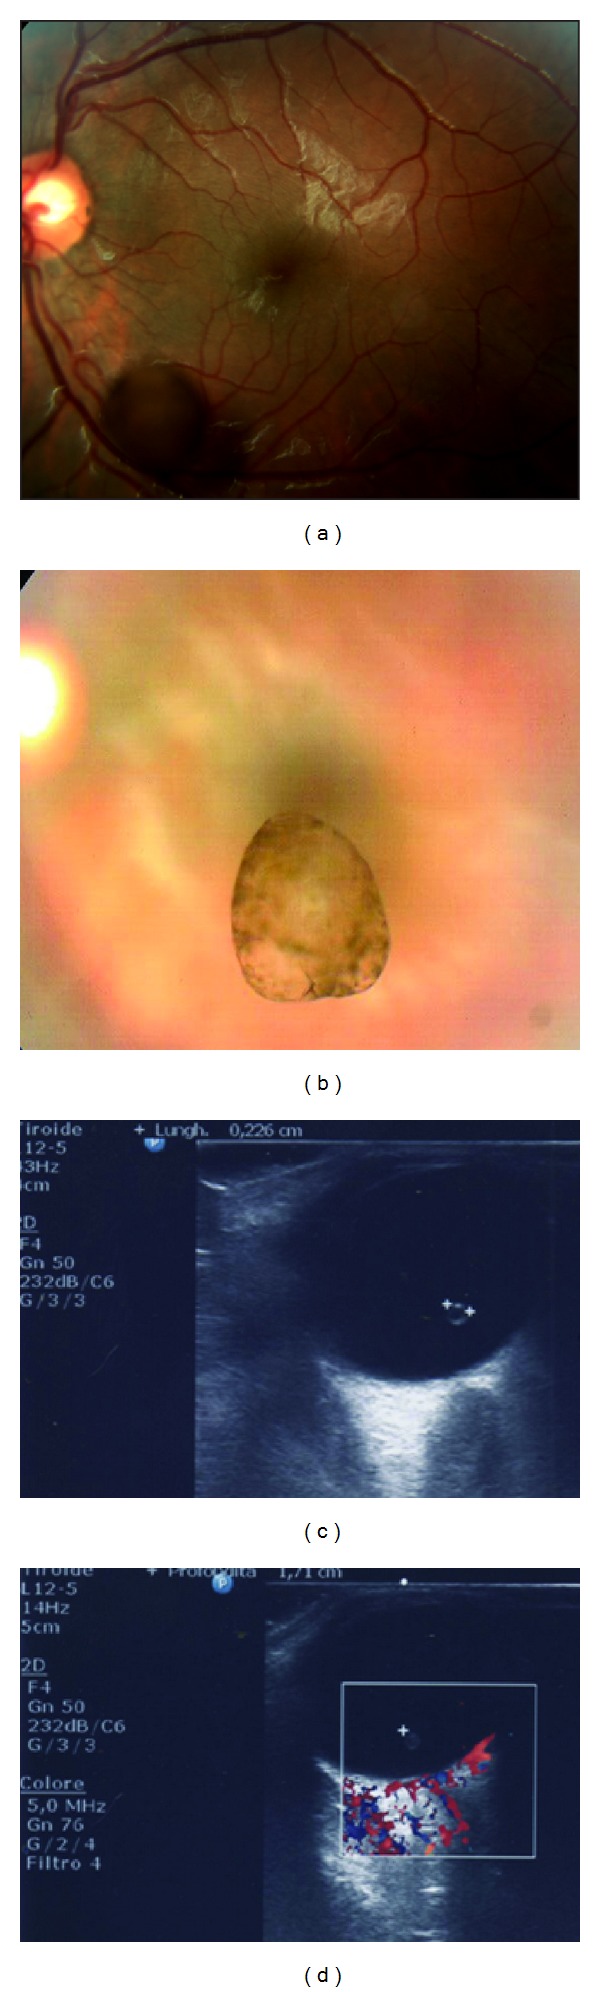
Ophthalmoscopy of the left eye shows a normal macula and a pigmented, oval-shaped cyst floating in the vitreous cavity and covering retinal vessels (a). The free-floating cyst was translucent, with a smooth brown-pigmented surface, and it was in the posterior vitreous (b). An oval-spherical hypoechogenic mass with thin hyper-reflective edges, with a 2.6-mm maximal diameter, was detected by real-time B-scan ultrasound of the left eye. The cyst was mobile in the posterior vitreous (c). Axial ultrasound with color Doppler showed no remnants of the hyaloid artery and no vessels in the vitreous of either of the globes, nor within the cyst (d).
